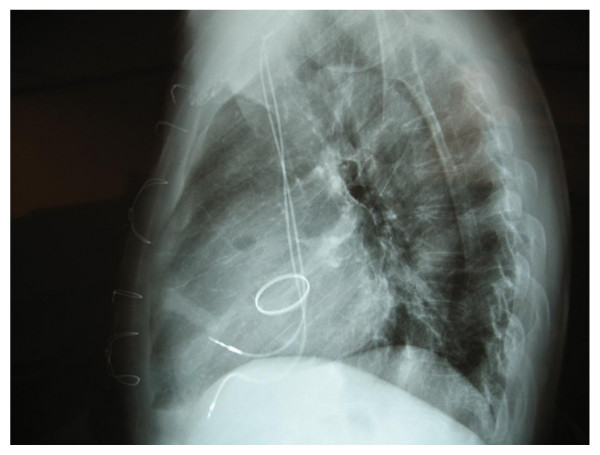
Post-operative chest X-ray after successfully performed Bentall- and Pacemaker-implantation.
